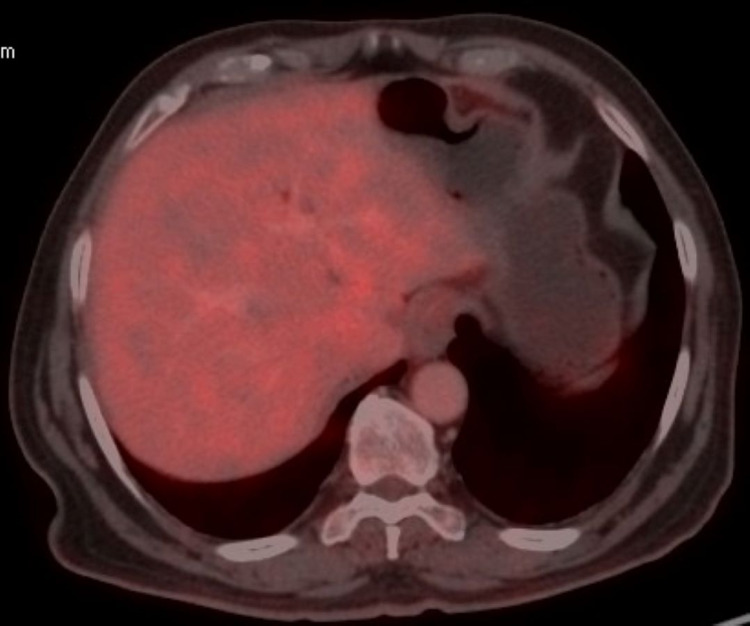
PET-CT scan after four cycles of pembrolizumab showing complete metabolic response in liver and stomach. PET-CT, positron emission tomography-computed tomography.
